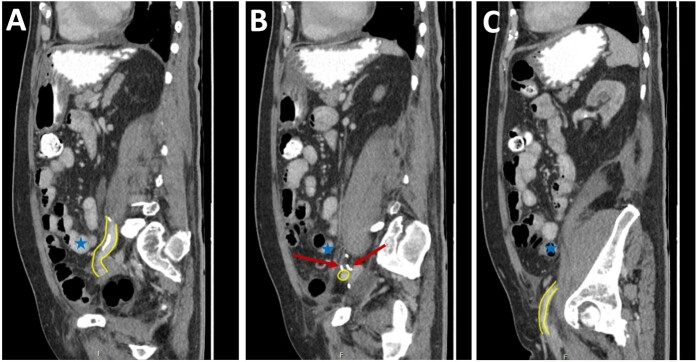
Post-operative CT scan. Series of sagittal images progressing from most medial (image A) to most lateral (image C). The patent EIA has been highlighted in yellow. Surgical clips are noted in image B (red arrow). Normal small bowel is noted in all images (blue stars).
